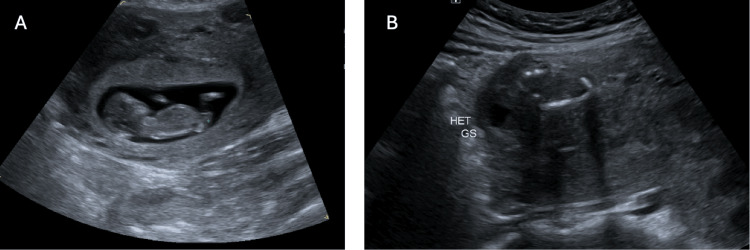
Pelvic ultrasound 21 days post-KCl administration (A) Progressive intrauterine pregnancy that is approximately 11+4 weeks gestation by crown rump length. (B) Previous right cornual ectopic pregnancy with nil further growth (approximately 1.7 x 0.9 x 1.1 cm).
